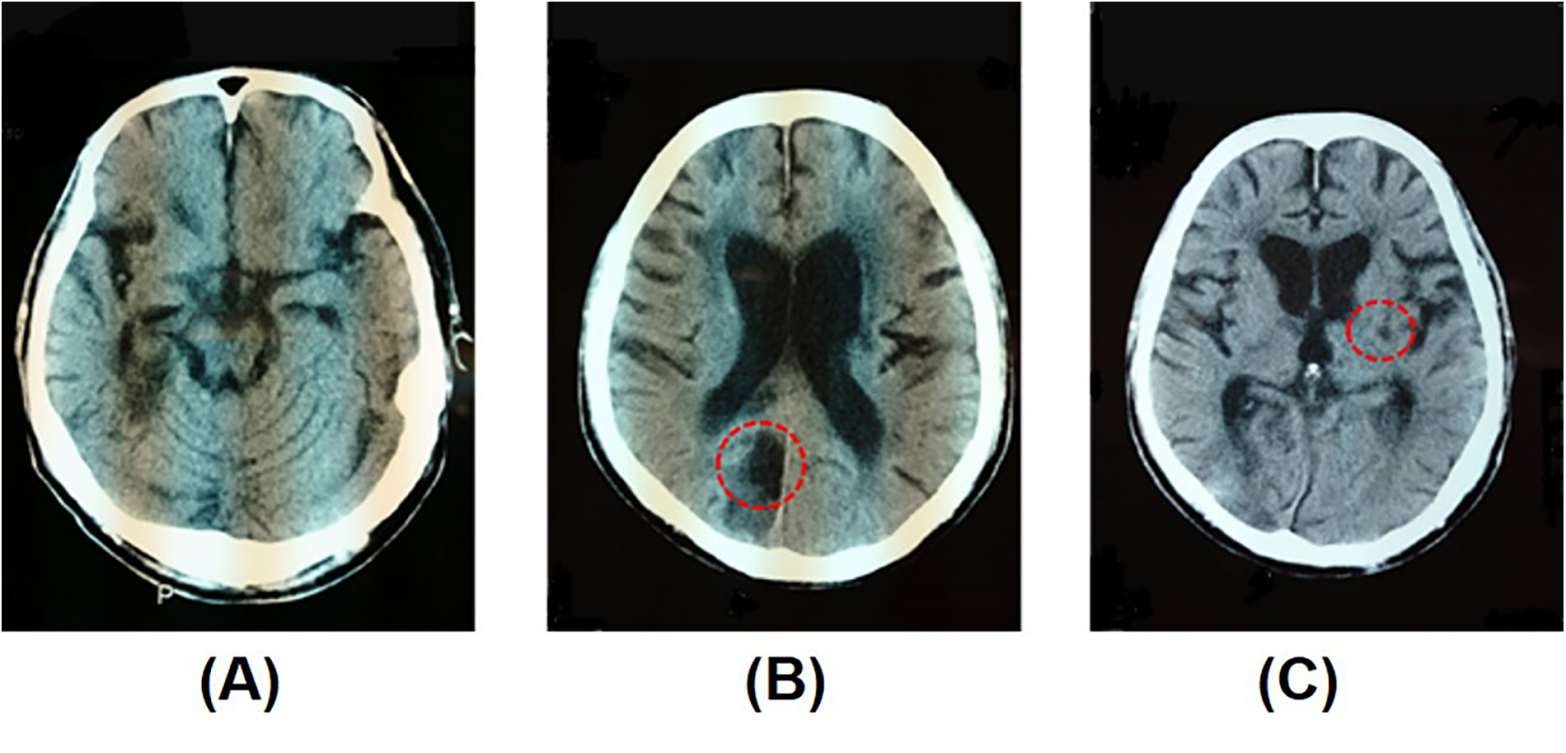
Brain computed tomography (CT) after the patient's third stroke. (A) No significant atrophy of the cerebrum, (B) infarction of the medial right occipital lobe, and (C) infarction of the left genu of the internal capsule (red circles).
